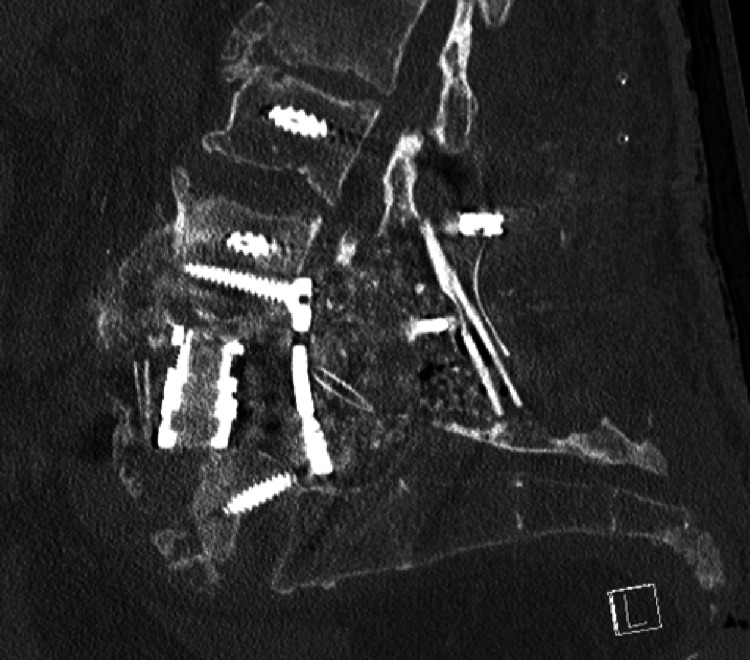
Sagittal CT of the lumbar spine demonstrating construct with an expandable cage, a lateral plate placed posteriorly, pedicle screw instrumentation, and an allograft strut placed posteriorly
